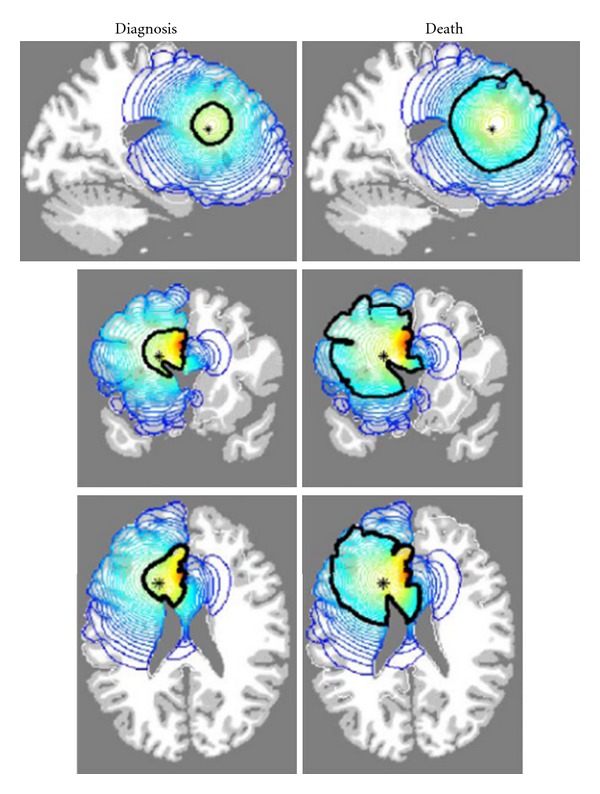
The left column corresponds to the tumour at diagnosis and right column corresponds to tumour at death. The dark black contour defines the detectable edge of tumour by (MRI), red contour indicates high density of tumour cells, and blue contour denotes low-density disease. Courtesy of Swanson et al. [2].
